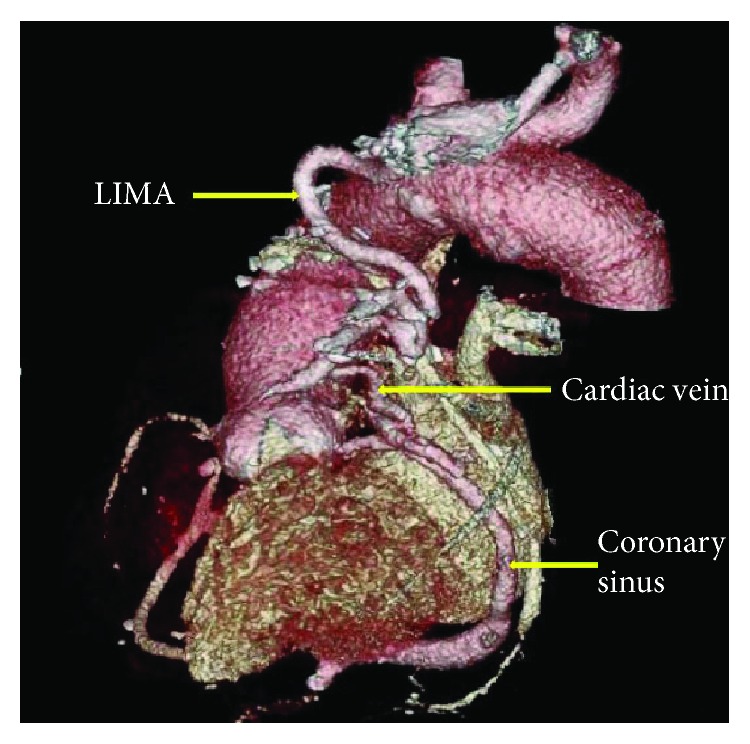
Cardiac computed tomography (CT) angiography with 3-dimensional reconstruction showed distal left internal mammary artery (LIMA) to left anterior descending (LAD) bypass artery communicating with a cardiac vein, which is eventually communicating with the coronary sinus, suggesting a coronary arteriovenous fistula.
